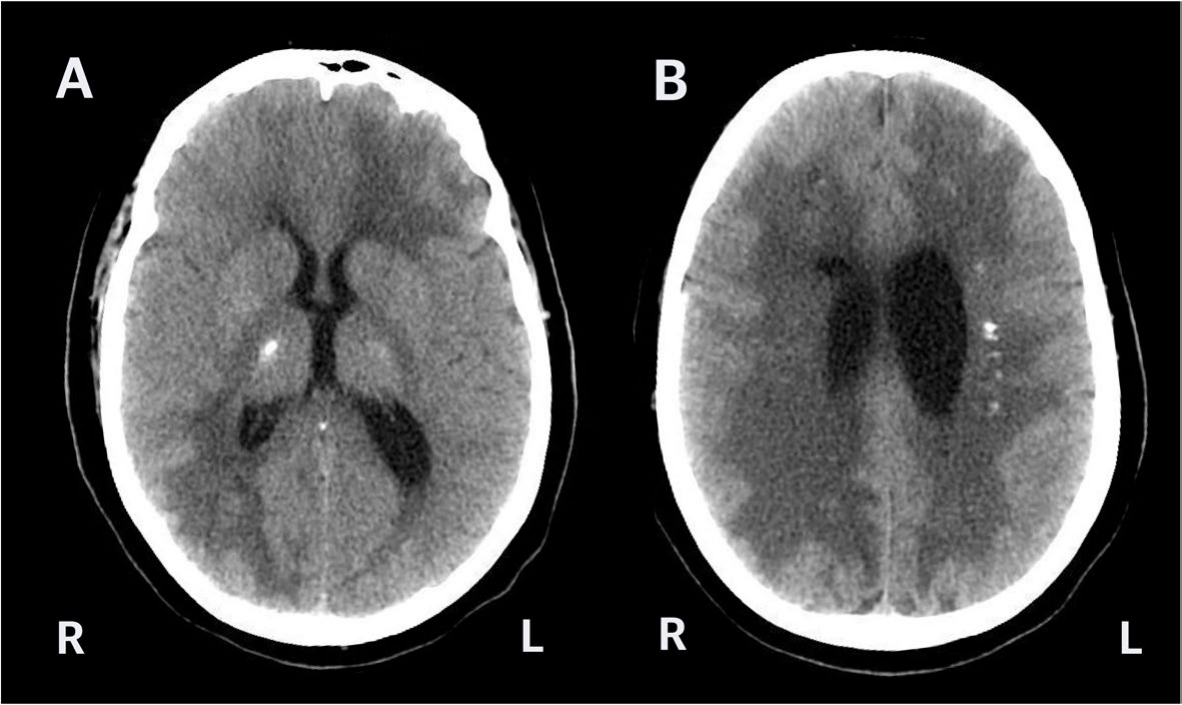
Computed tomography (CT) axial images. Calcifications were seen in the right thalamus (A) bilaterally within the cerebral white matter, here shown in the left centrum semiovale (B). The cerebral white matter also showed diffuse hypoattenuation (B)
